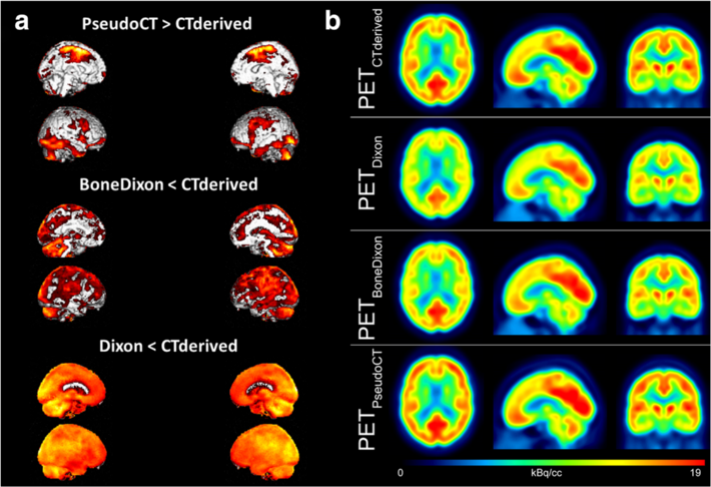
Comparison of different attenuation correction approaches in FDG brain PET/MRI. a Relative FDG uptake comparison by statistical parametric mapping between PET_PseudoCT/BoneDixon/Dixon_ vs. PET_CTderived_ from the 13 patients investigated (note: PET_BoneDixon_ was available in seven patients only). Midsagittal and lateral projections: significance level of *p* < 0.001; *T* value >3.9 (*T* > 5.2 for PET_BoneDixon_ due to lower sample size). Relative FDG uptake was significantly lower in PET_Dixon/BoneDixon_ as compared to PET_CTderived_ in a widespread area covering occipital, parietotemporal, and parieto-occipital cortices as well as the cerebellum. FDG uptake was significantly higher in PET_PseudoCT_ as compared to PET_CTderived_ in more restricted areas, including the primary and parietotemporal cortices as well as the cerebellum. b Mean images across all patients for the different attenuation correction approaches (after spatial normalization)
